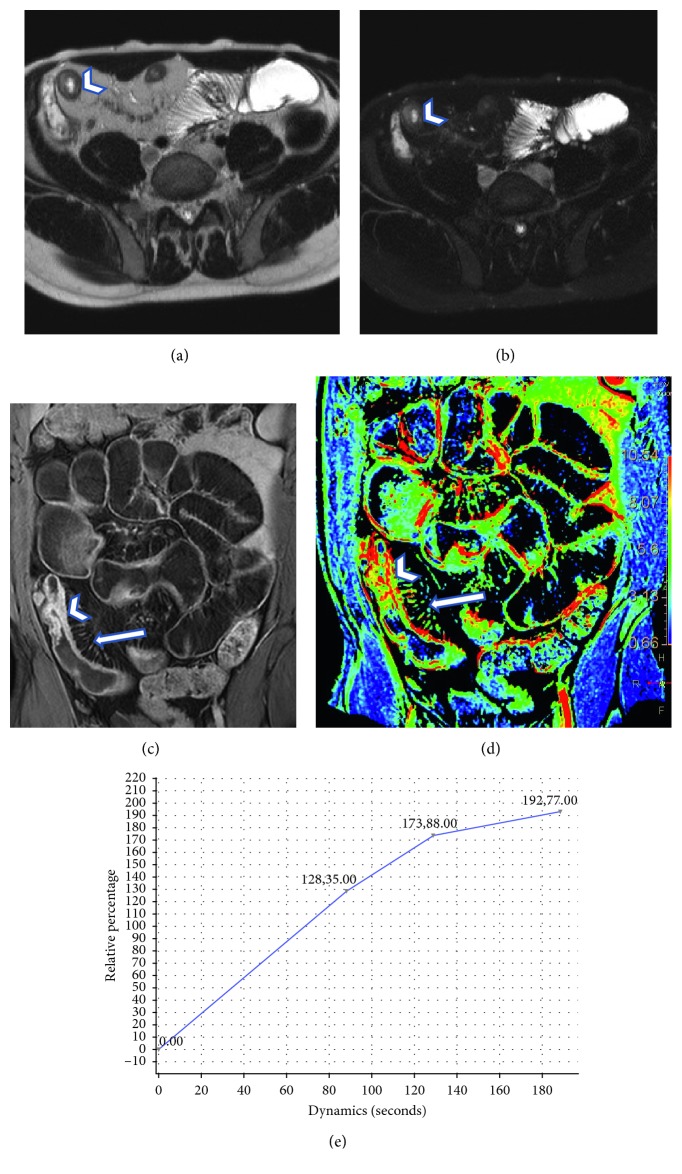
A 45-year-old man with known active disease characterized by the presence of mural thickening of last ileal bowel loop characterized by mild hyperintensity in the T2-weighted images (arrowhead in (a) and (b)), layered enhancement in dynamic study (arrowhead in (c)), and vascular congestion associated (arrow). The perfusion analysis allows highlighting the pathological segment in the color map (d), and the time-intensity curves demonstrate an increasing trend of enhancement for pathological loops.
